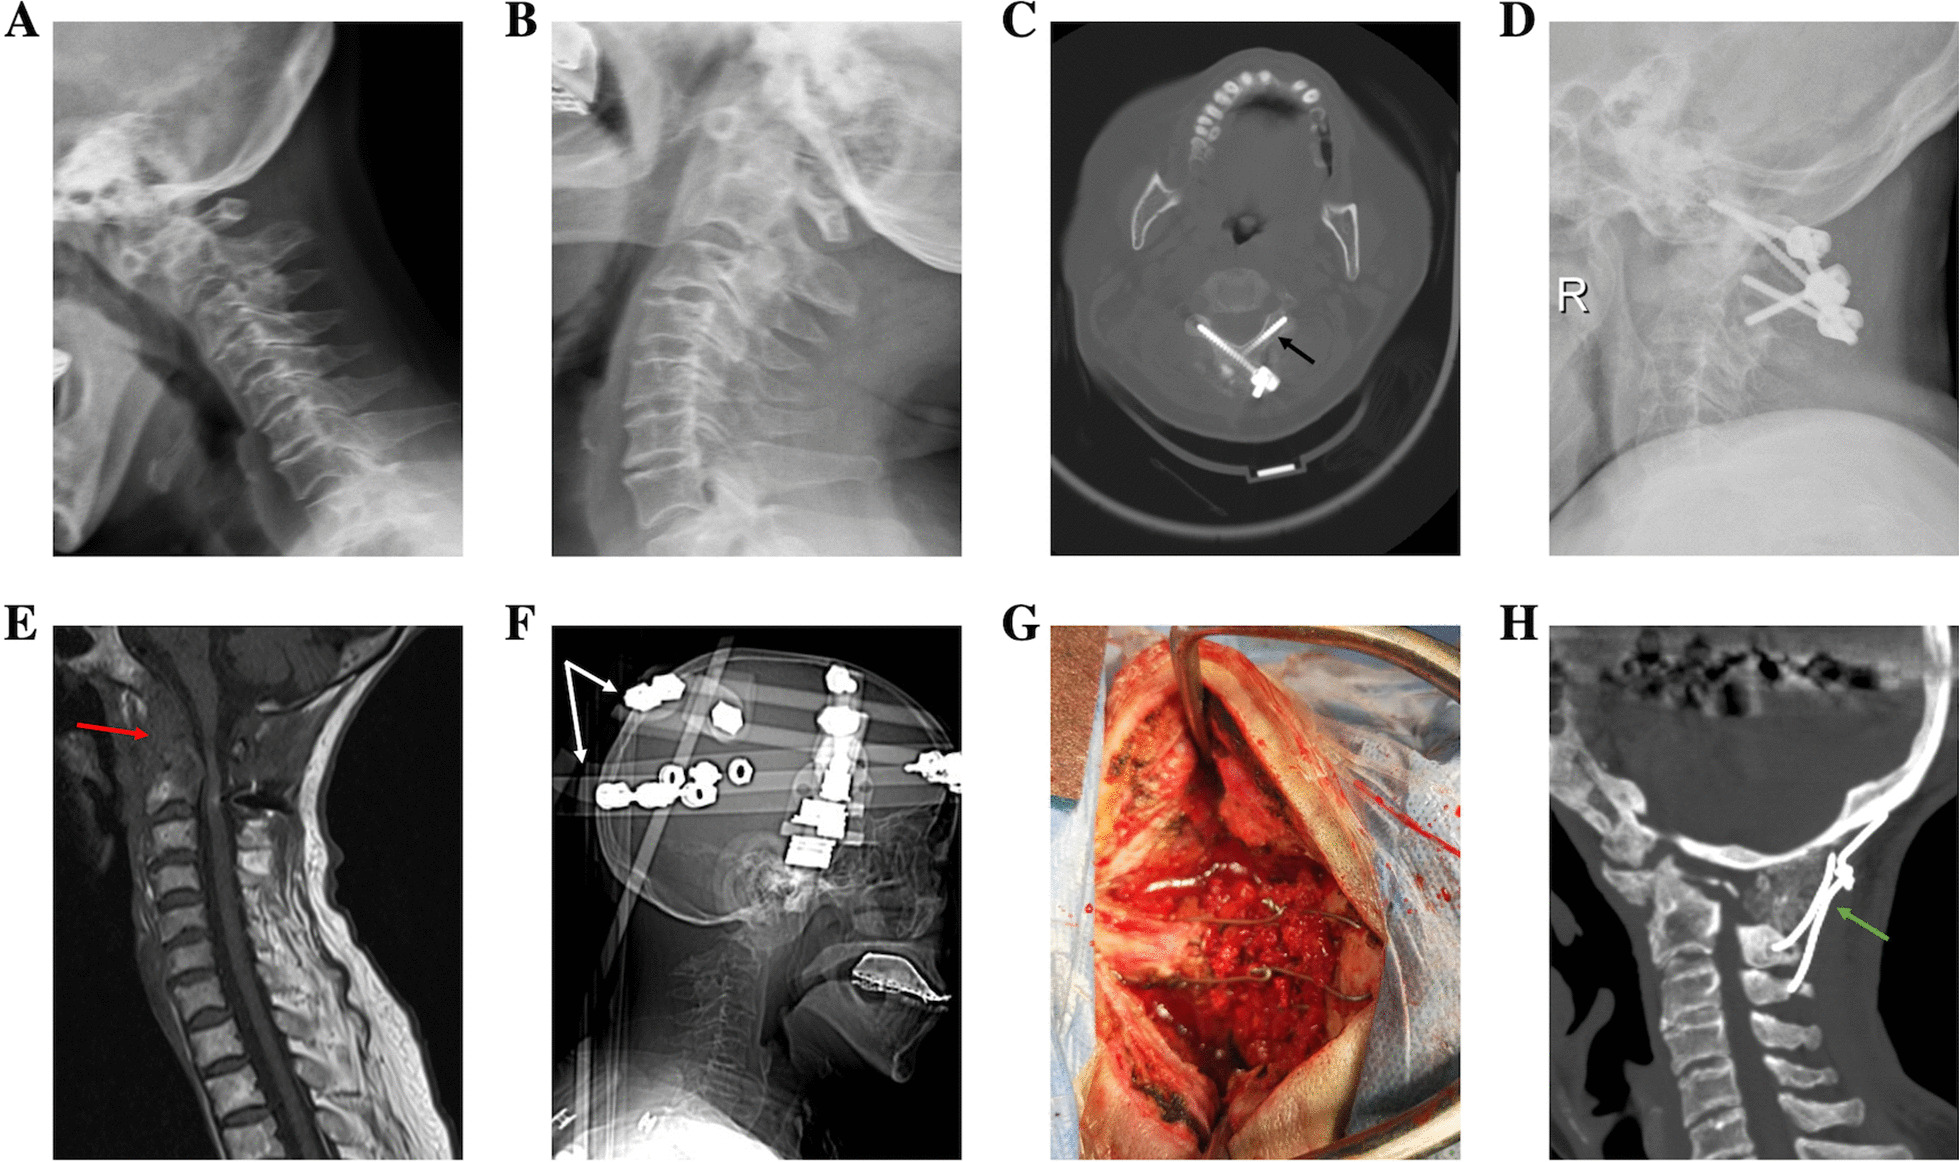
A 64-year-old female patient was admitted for weakness of both legs and walking instability for 9 months. Preoperative dynamic radiographs showed failed reduction of the atlantoaxial dislocation in a hyperextended or hyperflexed position (a, b), and after intraoperative traction, the atlantoaxial dislocation could still not be reduced, so it was classified as type III. Due to the high-riding vertebral artery, laminar screw fixation of the axis was performed (c, black arrow). At 2 months after operation, infection occurred (e, red arrow), and the internal fixation loosened and dislocated again (d). Debridement and anti-infection treatment were performed, the internal fixation was removed, and the head-ring brace was fixed (f, white arrow). Due to loosening of the original screw track, occipitocervical fusion was performed after infection control (g). The internal fixation position was acceptable at 3 months after operation, and bone graft healing was almost achieved (h, green arrow)
